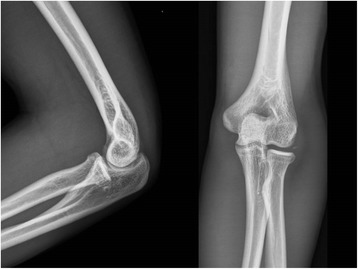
Plain radiographs failed to identify soft tissue injury and elbow instability
